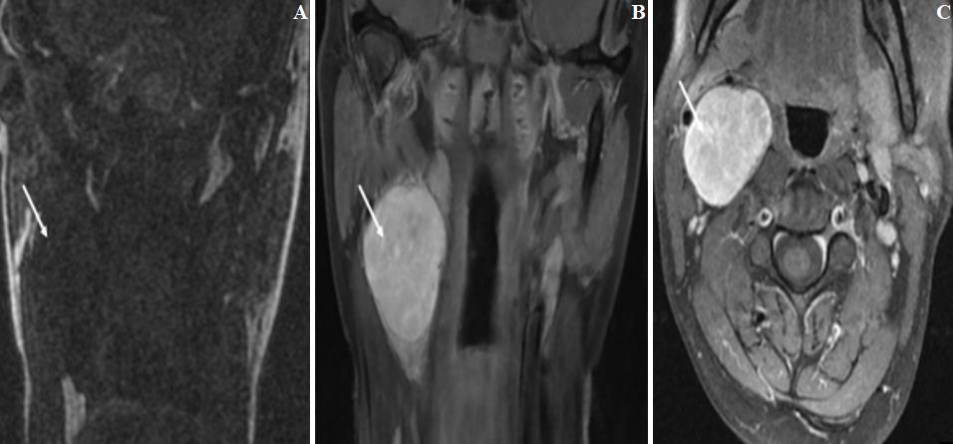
MR images showed masse was located later to the right submandibular region (arrow), homogeneous hypo intensity on T1-WI (A), heterogeneous hyper intensity on T2-WI (B), and heterogeneous enhancement on contrast-enhanced T1-WI (C)
